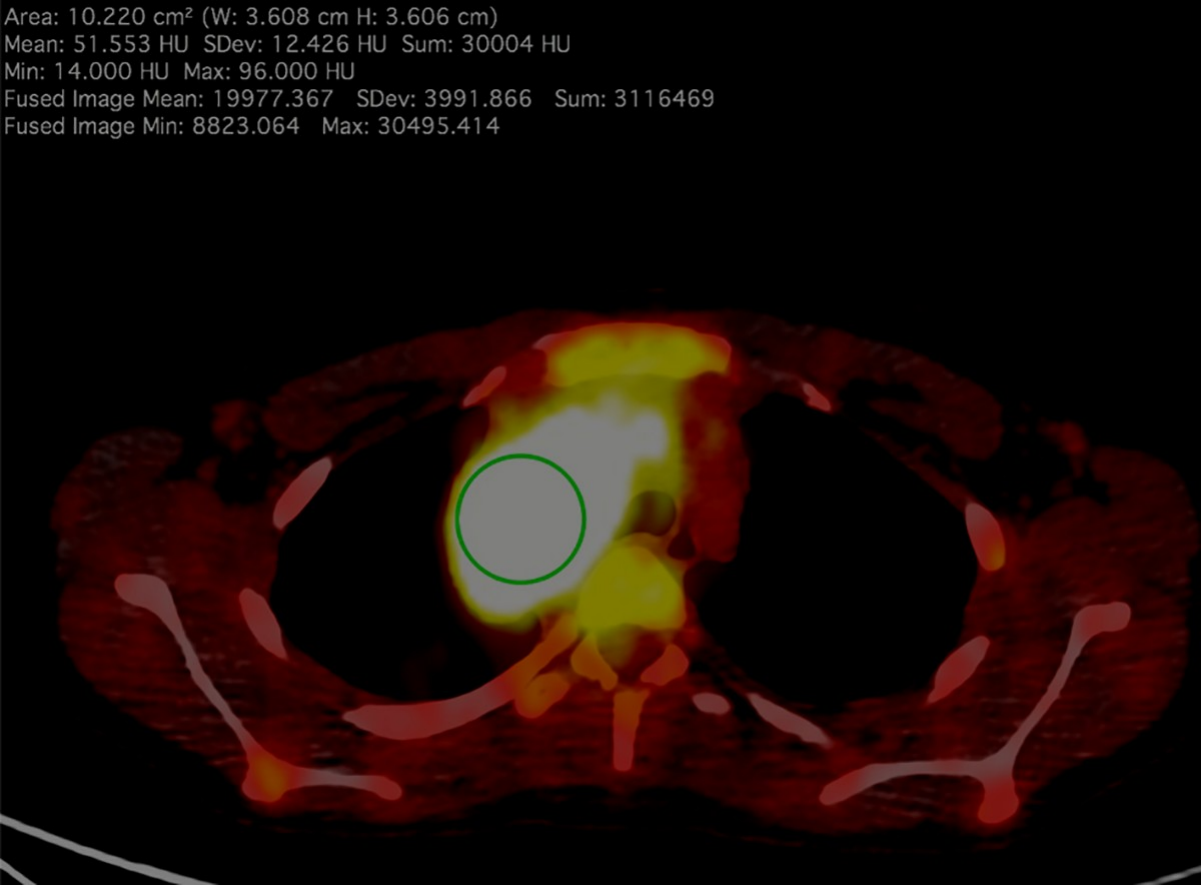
Fused PET-CT image of a female adolescent with HL; the measurement shows the highest value of both SUV_max_ (30.5) and XRA_max_ (96.0). The arithmetic mean of XRA_max_ was a subject to the statistical analysis.
